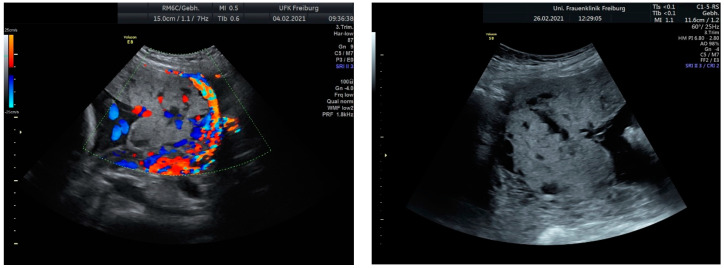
Irregular and large lacunae: Sonographic imaging reveals irregularly shaped and enlarged lacunae within the placenta. Hypervascularity: Increased vascularity is observed within the placenta, indicating abnormal blood flow. Turbulent flow in lacunae: Doppler imaging demonstrates turbulent blood flow within the lacunae, suggestive of abnormal vascularization. Changes in diameter: Variations in the diameter of lacunae may be noted on sonographic examination, reflecting the dynamic nature of placental vascularization in PAS.
